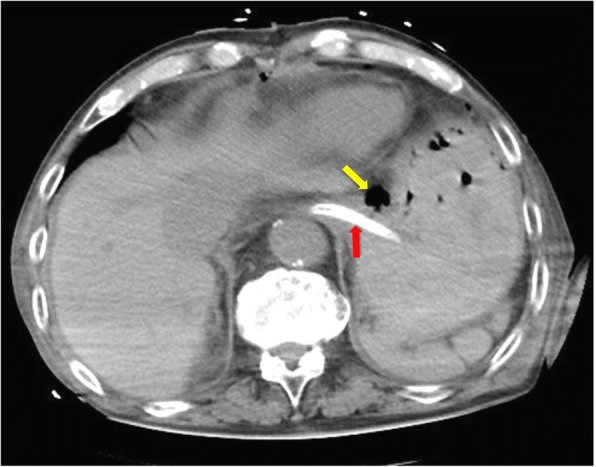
Computed tomography image on postoperative day 1, showing localized free air in the abdominal cavity. The yellow arrow indicates an area of free air between the left lateral segment of the liver and the lesser curvature of the stomach. The red arrow indicates the nasogastric tube from the lower esophagus to the cardia
